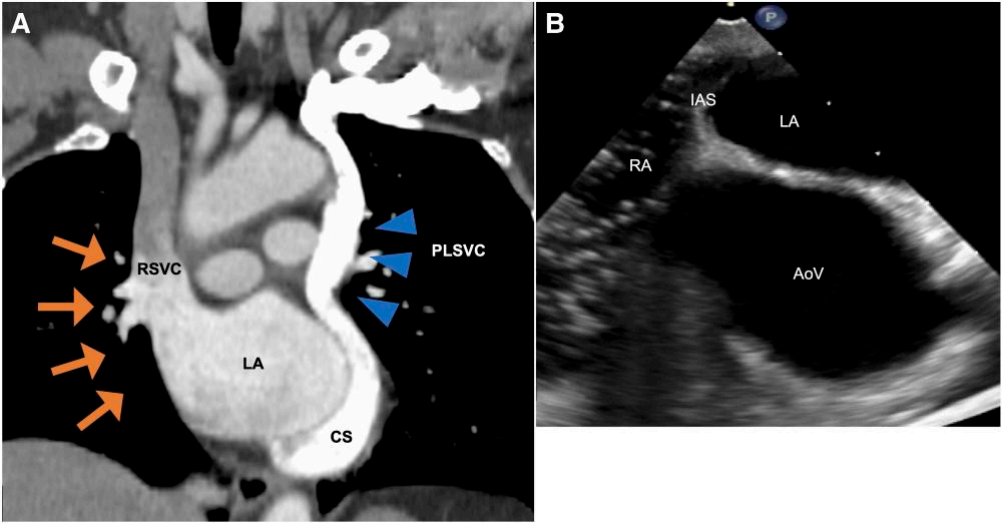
Contrast-enhanced CT reveals RSVC drainage into the LA (arrows) with PLSVC (arrowheads) and the absence of connection between CS and LA (*A*). Transoesophageal echocardiographic examination with a microbubble test injected via the left median cubital vein confirms the lack of a connection between the CS and LA (*B*). RSVC, right superior vena cava; LA, left atrium; PLSVC, persistent left superior vena cava; CS, coronary sinus; CT, computed tomography; RA, right atrium; IAS, interatrial septum; AoV, aortic valve.
